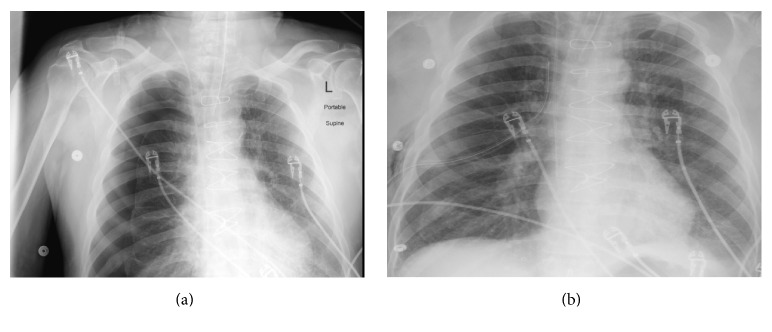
(a) Chest X-Ray showing large right-sided pneumothorax with lung margin collapse more than 4 cm from the chest wall uniformly. (b) Chest X-Ray showing Interval placement of chest tube with tip in the right suprahilar area with near-complete lung reexpansion.
